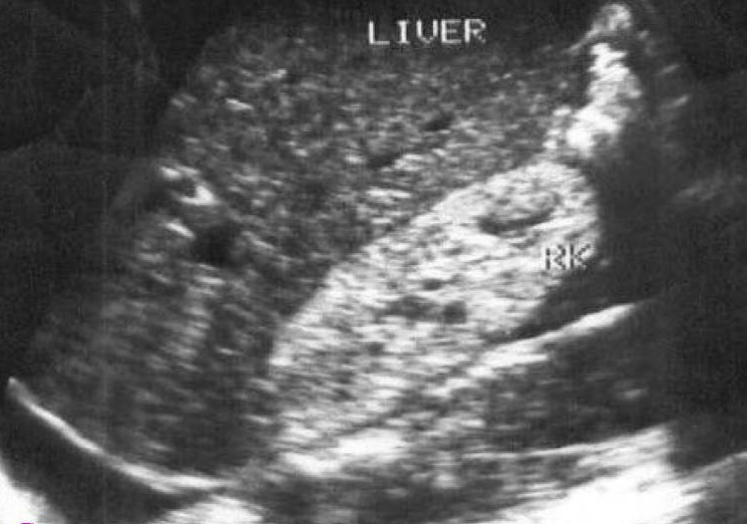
HIV nephropathy in a 34-year-old man. Longitudinal sonogram demonstrates normal sized right kidney with increased cortical echogenicity. Diffuse increased cortical echogenicity is associated with a poor prognosis.
